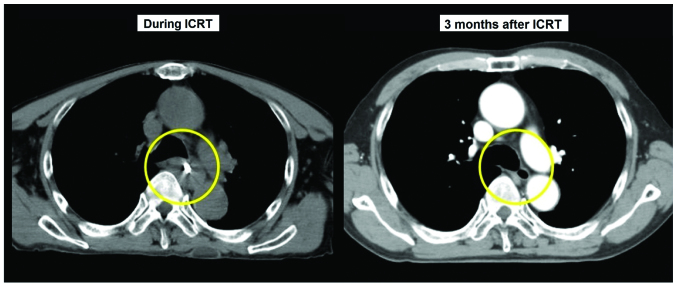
Computed tomography scan indicating a marked improvement in esophageal wall thickness at three months post-ICRT compared with during ICRT. ICRT, intracavitary radiation therapy.
